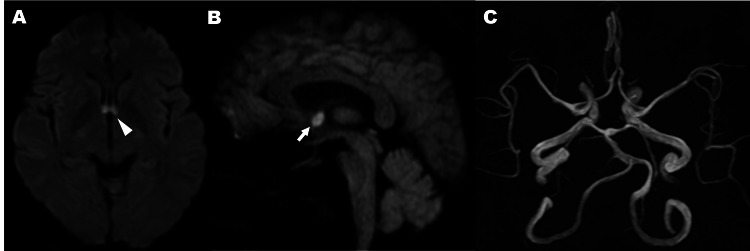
MRI findings at the time of admission A: Axial diffusion-weighted imaging (DWI) on day 0 shows bilateral fornix infarction, indicated by a white arrowhead; B: Sagittal DWI from the same day reveals that the infarct is confined to the column of the fornix (white arrow); C: Caudal view of the MRA demonstrates no stenosis or occlusion in the major cerebral arteries, particularly the anterior cerebral artery. The magnified inset in the lower right shows the anterior communicating artery. MRA: Magnetic resonance angiography
